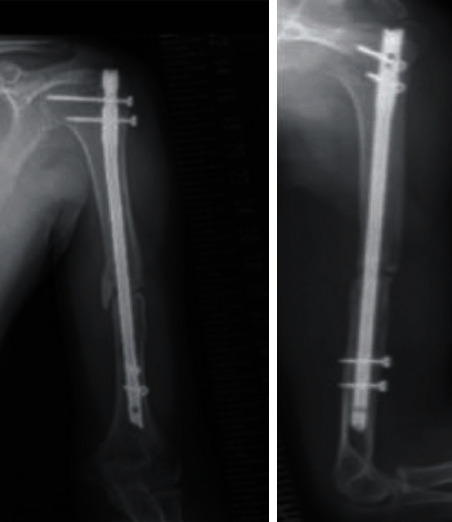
Radiographs at the first visit to our hospital. Nonunion was observed with callus formation on the medial side.
